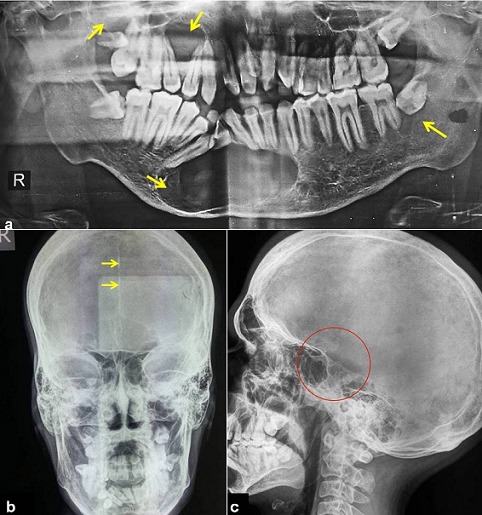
a) orthopantomogram showing multiple jaw cysts; b) posterio-anterior and lateral view of the skull showing calcification of falx cerebri; c) bridging of sella turcia
